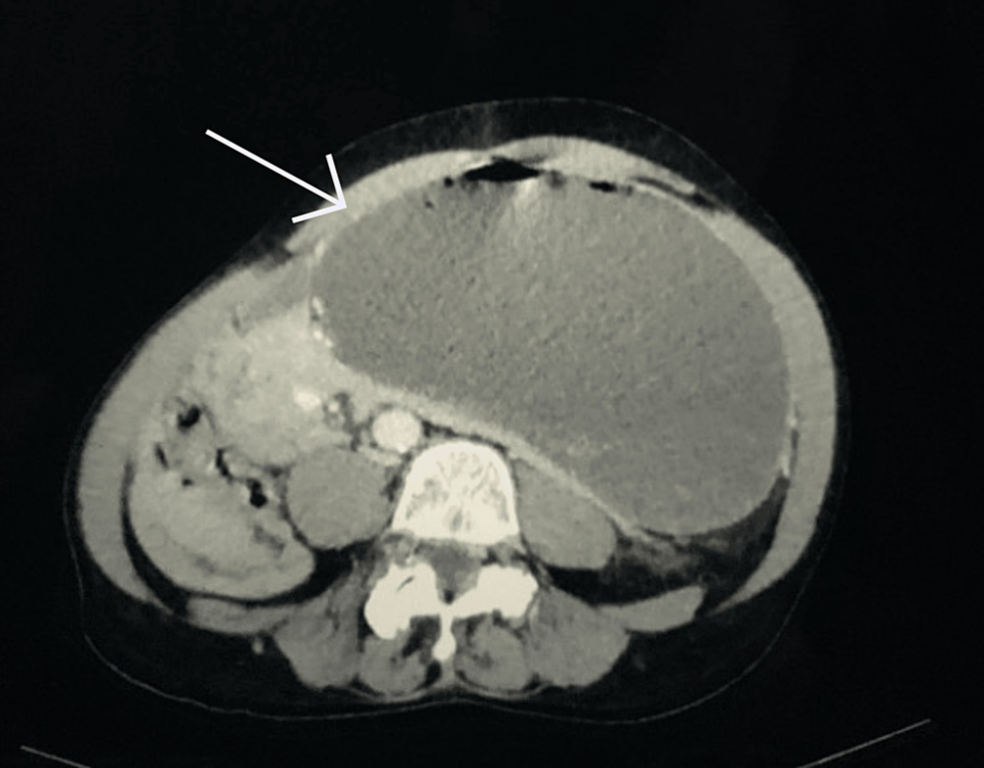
Computed tomography of abdomen and pelvis showing dilated stomach extending into the low pelvis. Arrow pointing to the dilated stomach extending into the low pelvis.
